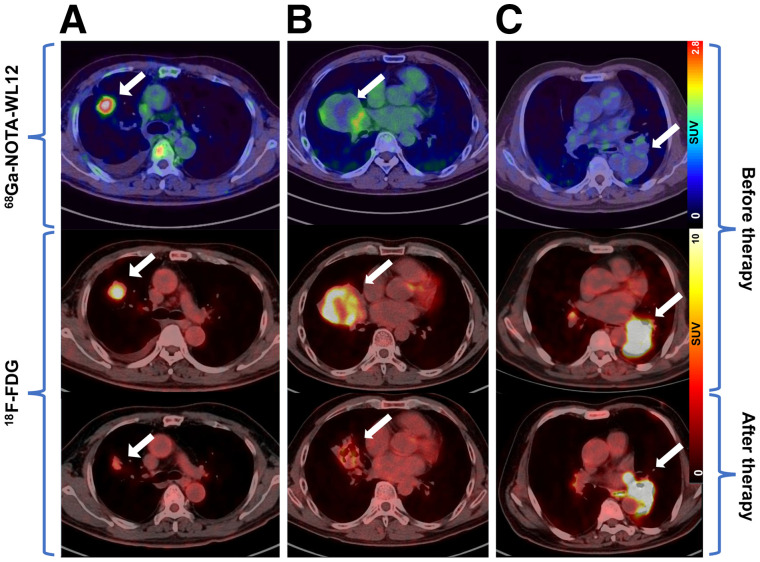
(A) Patient 6, a 58-y-old man with adenocarcinoma and a PD-L1 TPS of 30%. SUV_max_ of ^68^Ga-NOTA-WL12 was 3.05 and that of ^18^F-FDG decreased from 8.03 to 3.10. (B) Patient 1, a 47-y-old man with squamous cell carcinoma and a TPS of 8%. SUV_max_ of ^68^Ga-NOTA-WL12 was 2.21 and that of ^18^F-FDG decreased from 9.13 to 3.54. (C) Patient 3, a 68-y-old man with squamous cell carcinoma and a TPS of 8%. SUV_max_ of ^68^Ga-NOTA-WL12 was1.84 and that of ^18^F-FDG increased from 16.55 to 21.38. All lesions were indicated by white arrows.
